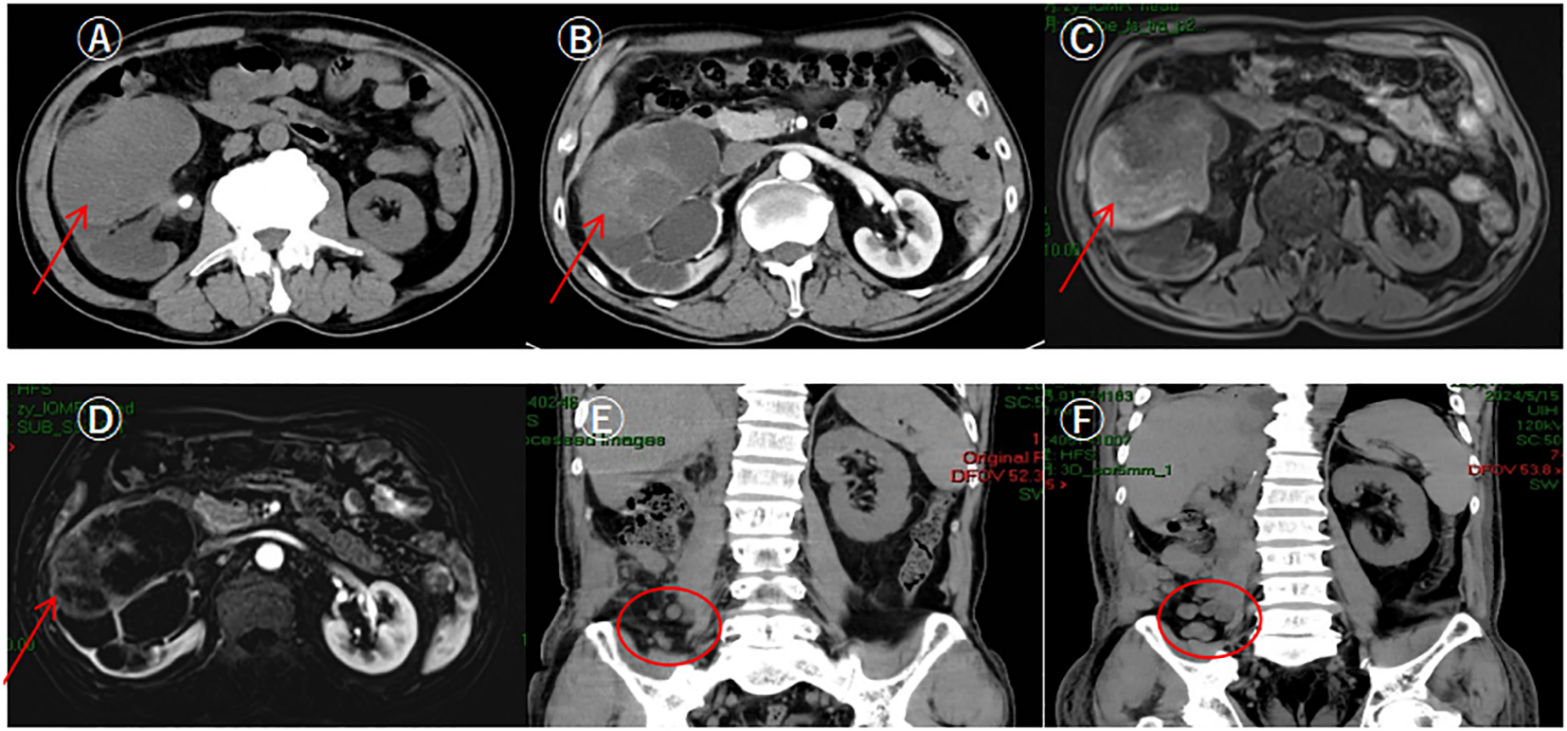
Patient's preoperative CT urography, upper abdominal MRI and postoperative upper abdominal CT. (A) CTU showed a huge soft tissue mass shadow in the middle part of the right kidney, with clear margins of the mass, and its inner density was uneven (pointed out by arrows); (B) CTU enhancement scan showed a mild uneven delayed enhancement, with a slight thickening of the neighboring fascia, and gross; (C) MRI showed a mass shadow in the mid-pole of the right kidney, with an uneven inner signal, and clear boundaries; (D) MRI enhancement scan showed uneven enhancement; (E) Review CT on the 26th day after surgery showed multiple round-like soft tissue nodules in the right iliac fossa, with a diameter of about 1.0 cm, and lymph node metastasis was considered; (F) Review CT on the 37th day after surgery showed multiple nodular and irregular mass-like soft tissue density shadows in the operated area and the right posterior abdominal wall and the right iliac fossa, with the foci enlarging and increasing in size compared with the previous ones, and lymph nodes were considered to be multiple metastasis.
